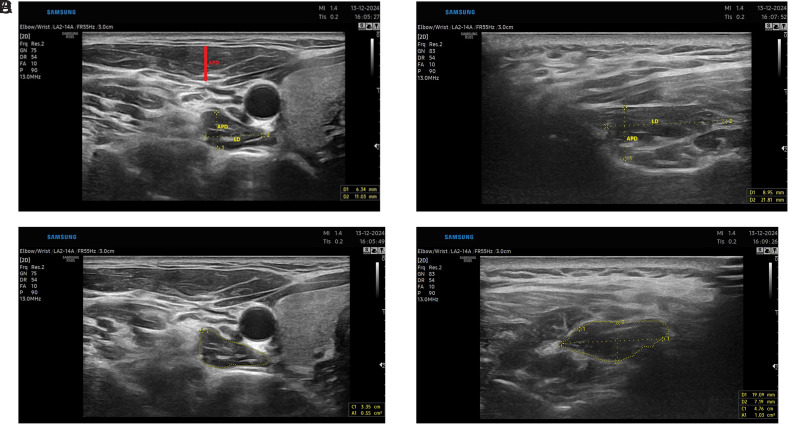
Ultrasound imaging of muscles. A) Dashed lines show the anteroposterior dimension (APD) and lateral dimension (LD) of the longus colli. The solid line shows the anteroposterior dimension of the sternocleidomastoid. B) Dashed lines show the anteroposterior dimension (APD) and lateral dimension (LD) of the cervical multifidus. C) Cross-sectional area measurement of the longus colli is shown. D) Cross-sectional area measurement of the cervical multifidus is shown.
